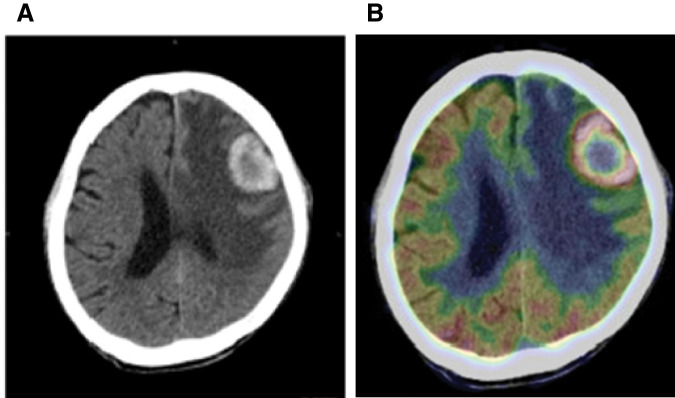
Imaging findings at the time the brain tumor was identified (A) Plain CT showing a neoplastic lesion in the left frontal lobe with hemorrhage and surrounding edema. (B) PET-CT showing an abnormal accumulation, with an SUVmax = of 10.1 around the margins of the mass. No other distant metastases or new lesions were noted.
